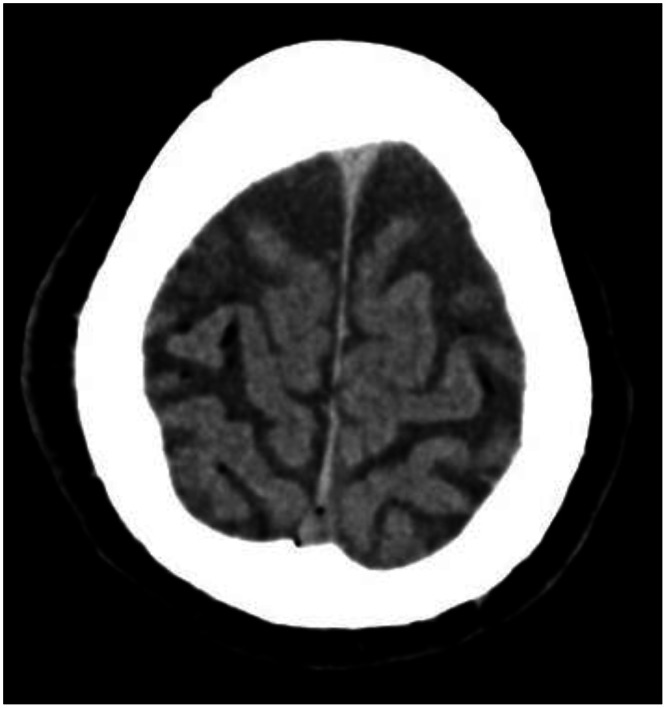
CT scan head pneumocephalus.
